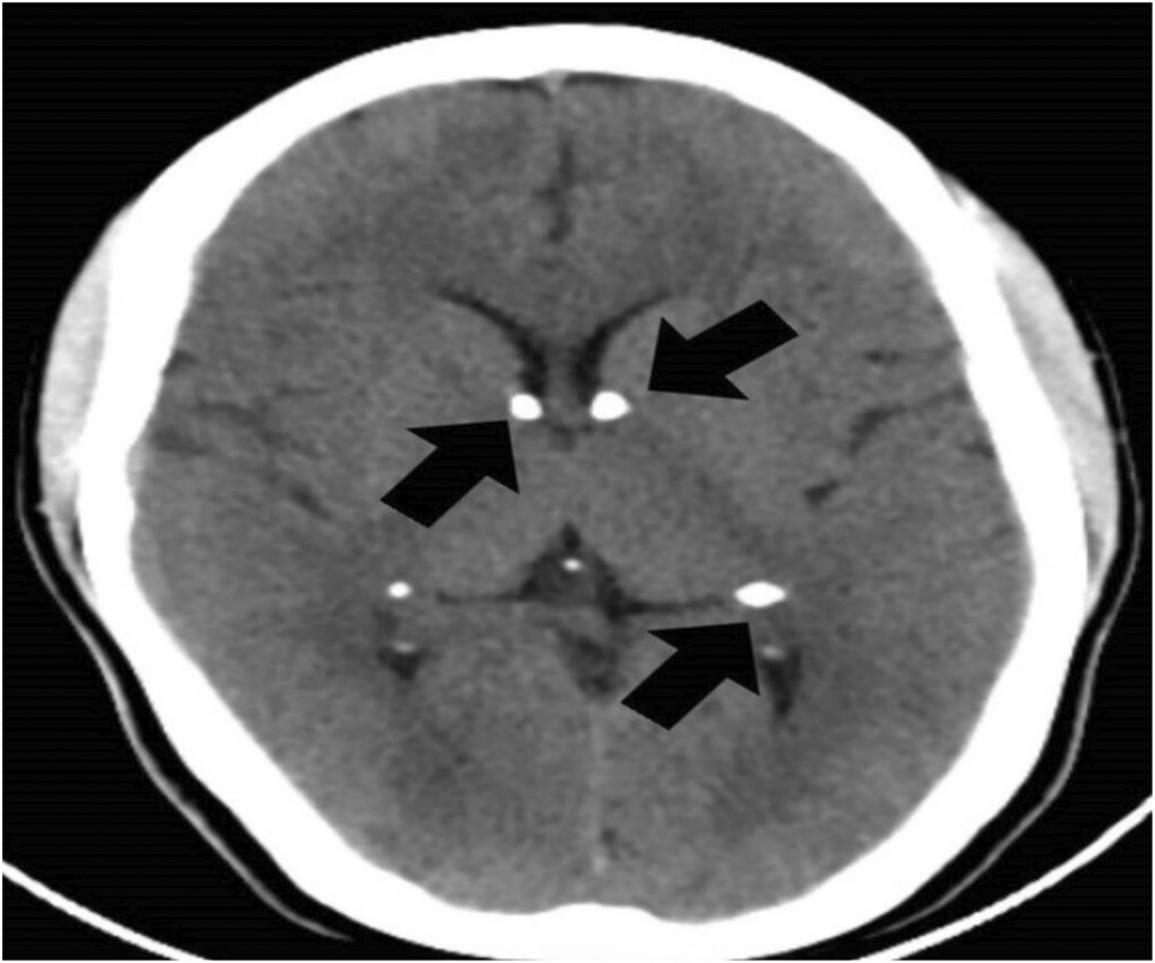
Axial computed tomography of the brain, calcified subependymal nodules along the margin of the ventricles (black arrows)
